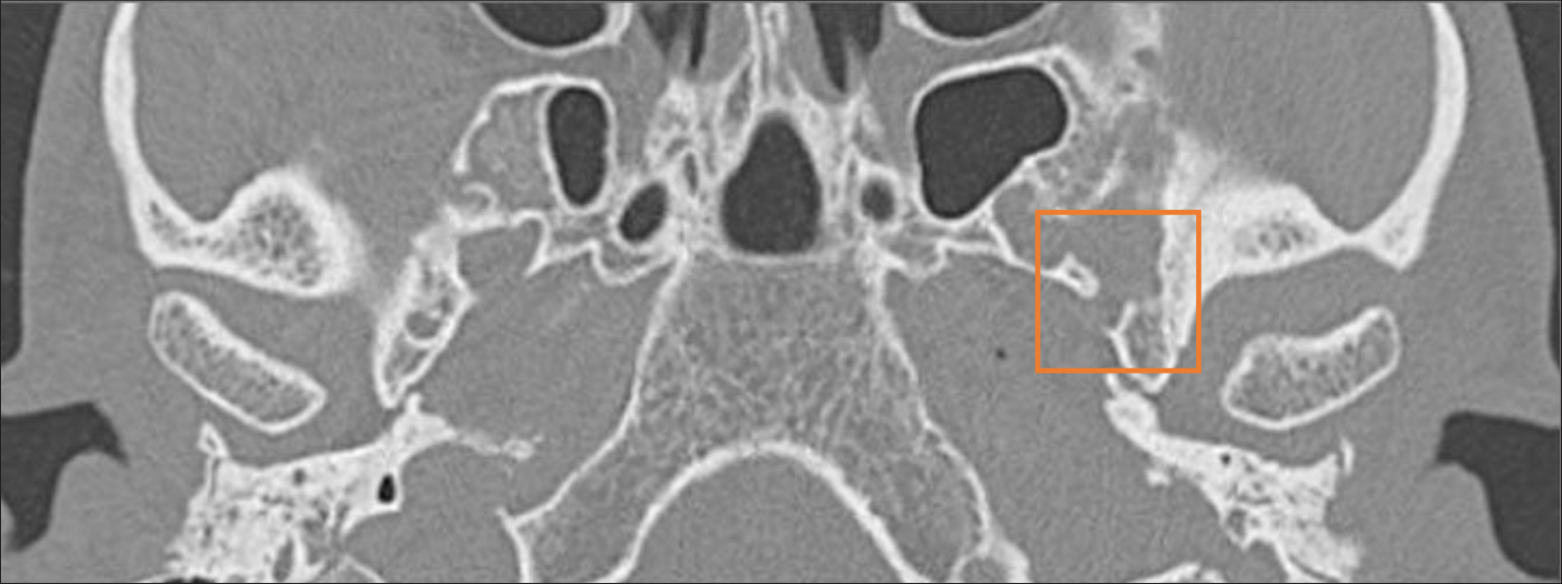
Axial CT scan showing the absence of the left foramen spinosum.
